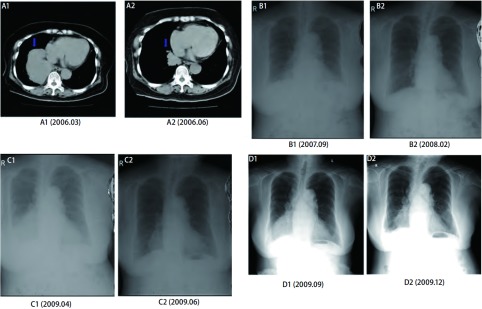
治疗前后CT及X光影像。A1：GP方案治疗前右肺肿瘤（9.6 cm×5.3 cm）；A2：GP方案治疗后右肺肿瘤（4.2 cm×2.2 cm）；B1：GP方案治疗前右肺肿瘤（3.0 cm×2.5 cm）；B2：GP方案治疗后右肺肿瘤几乎消失；C1：GP方案治疗前右肺肿瘤（7.0 cm×8.5 cm）；C2：GP方案治疗后右肺肿瘤（4.2 cm×5.7 cm）；D1：GP方案治疗后右肺肿瘤（6.7 cm×6.5 cm）；D2：GP方案治疗后右肺肿瘤（4.9 cm×6.1 cm）。 CT image and X-ray image before or after chemotherapy. A1: Tumor in right lung (9.6 cm×5.3 cm) before GP treatment; A2: Tumor in right lung (4.2 cm×2.2 cm) after GP treatment; B1: Tumor in right lung (3.0 cm×2.5 cm) before GP treatment; B2: Tumor in right lung (almost disappeared) after GP treatment; C1: Tumor in right lung (7.0 cm×8.5 cm) before GP treatment; C2: Tumor in right lung (4.2 cm×5.7 cm) after GP treatment; D1: Tumor in right lung (6.7 cm×6.5 cm) before GP treatment; D2: Tumor in right lung (4.9 cm×6.1 cm) after GP treatment.
